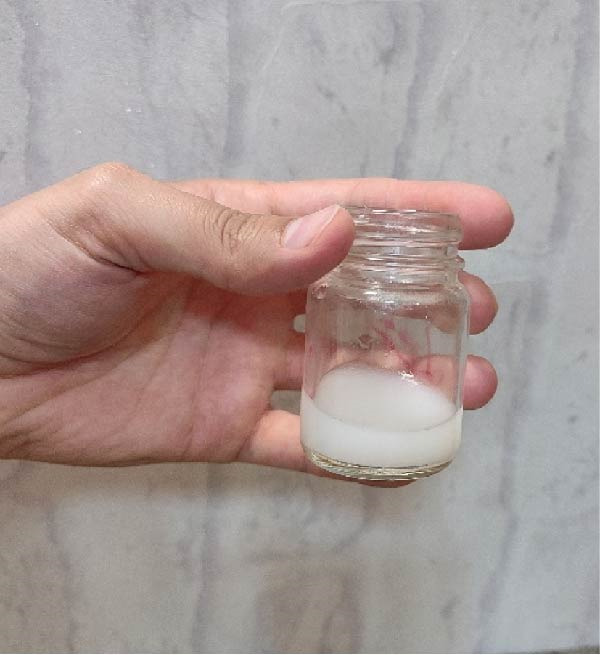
Homogeneous white suspension of green‐synthesized ZnO NPs dispersed in double‐distilled water after 20 min of ultrasonication.
